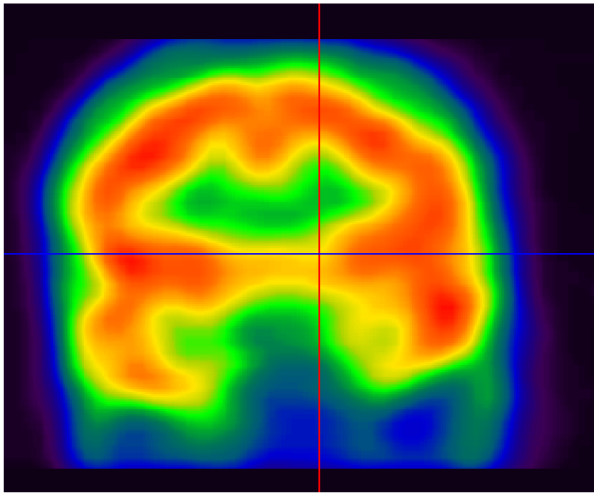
Pretreatment brain perfusion single-photon-emission computed tomography, showing low perfusion in the left temporal lobe.
